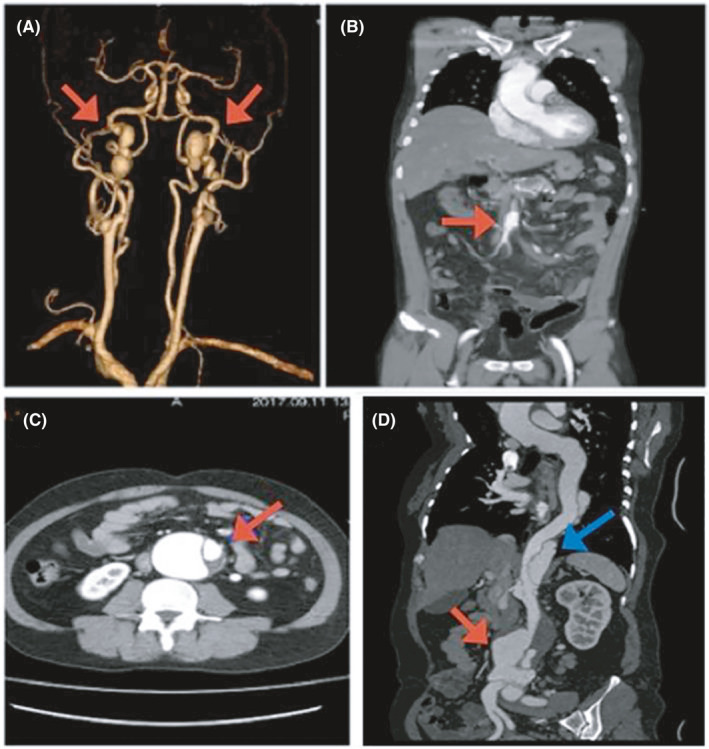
Vascular abnormalities in two patients with vascular Ehlers‐Danlos syndrome. (A) Coronal colour‐coded 3D volume‐rendered image showing bilateral internal carotid artery aneurysms (arrows) in the proband (III:9; see Figure 1). (B) Coronal contrast‐enhanced computed tomography angiography (CTA) showing a superior mesenteric artery dissection aneurysm (arrow) in the proband. (C) Axial contrast‐enhanced CTA showing type B aortic dissection (arrow) in the proband's 39‐year‐old elder sister (III:7; see Figure 1). (D) Coronal contrast‐enhanced CTA showing type B aortic dissection (blue arrow) and abdominal aortic aneurysm (red arrow) in III:7
